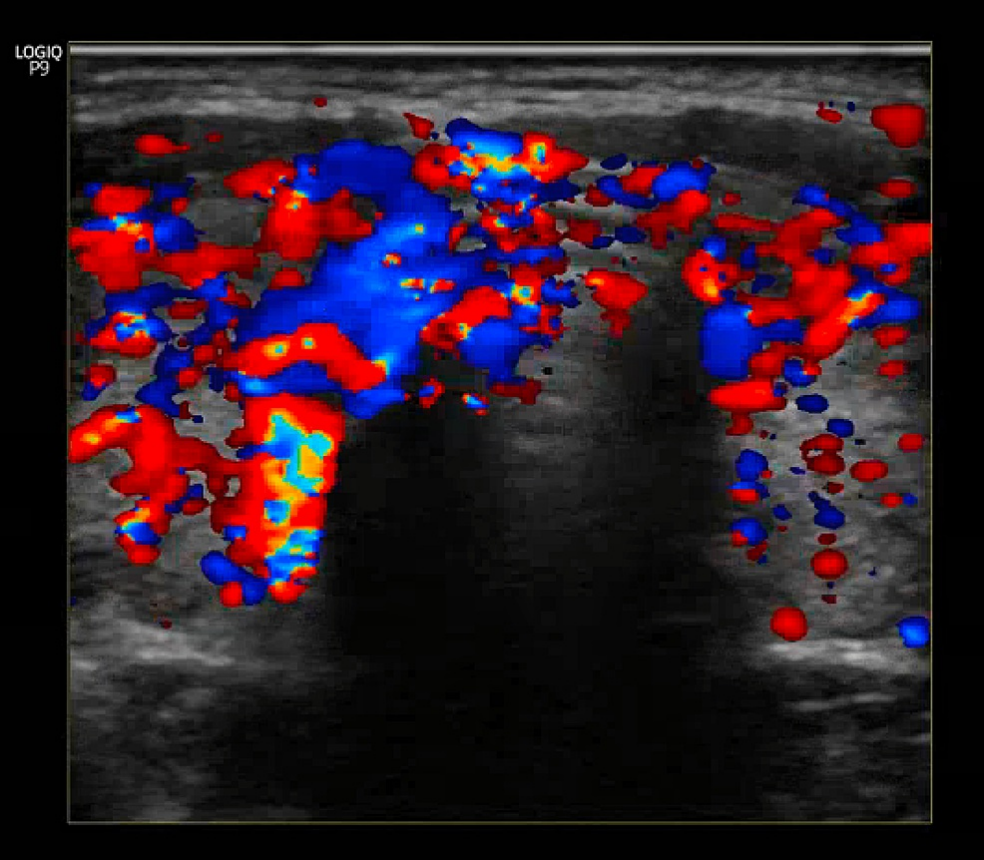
Ultrasound of the thyroid gland showing diffuse increased vascular flow throughout the gland
